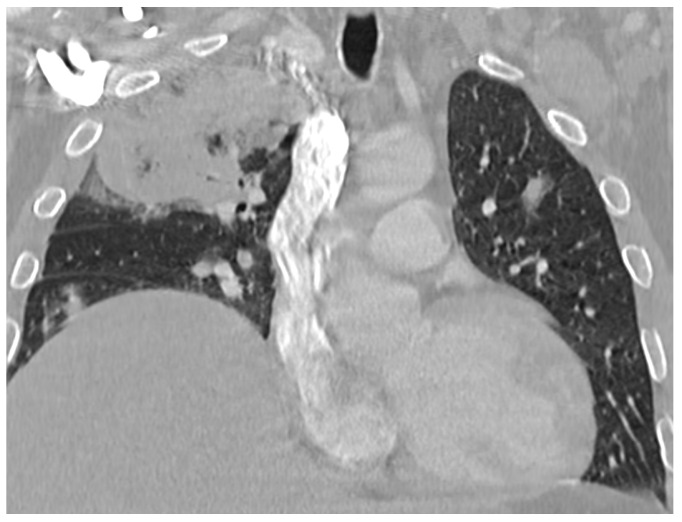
Chest CT opacity in a patient with prolonged immobilization and *Klebsiella pneumonia*: consolidation in the right upper lobe with slight bulging of the medial segment of the right horizontal fissure and surrounding ground-glass. Representative image from the anonymized institutional radiology archive of University Hospital Frankfurt.
